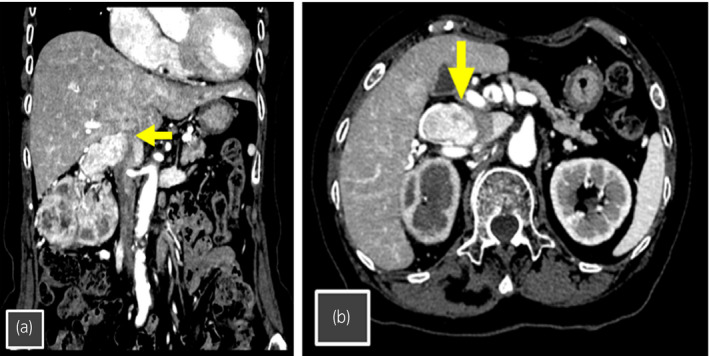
Computed tomography showing right RCC with a level 1 IVC tumor thrombus (arrow). (a) Coronal section. (b) Axial section.
